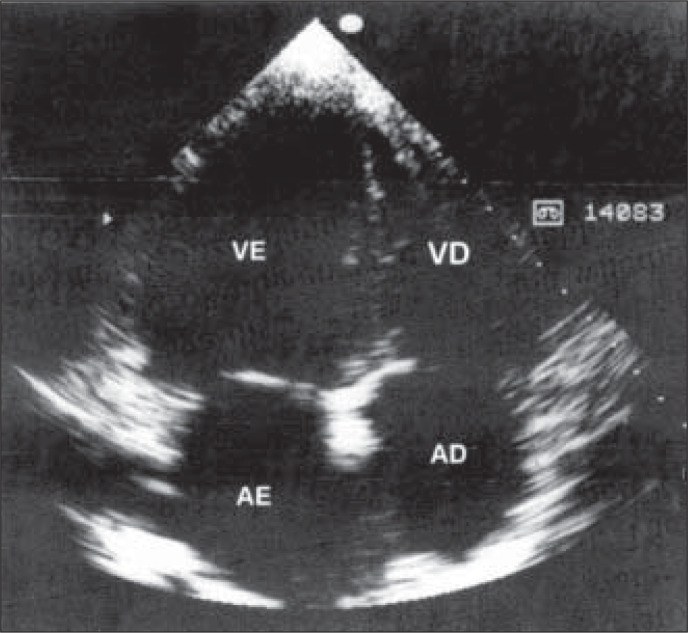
Two-dimensional echocardiogram in the 4-chamber view, showing general dilatation.
